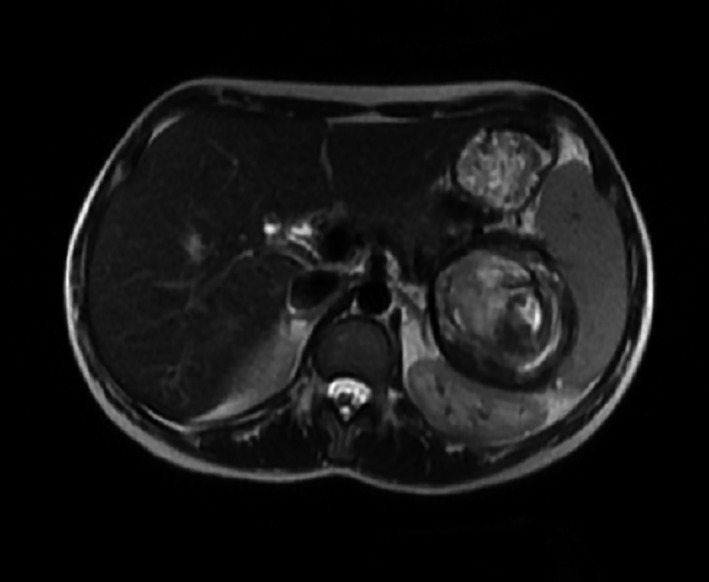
Axial cut on MRI showing a 7.5 × 7 × 6.5 cm aneurysm in the distal portion of the splenic artery with an intramural thrombus, abutting the splenic hilum.
